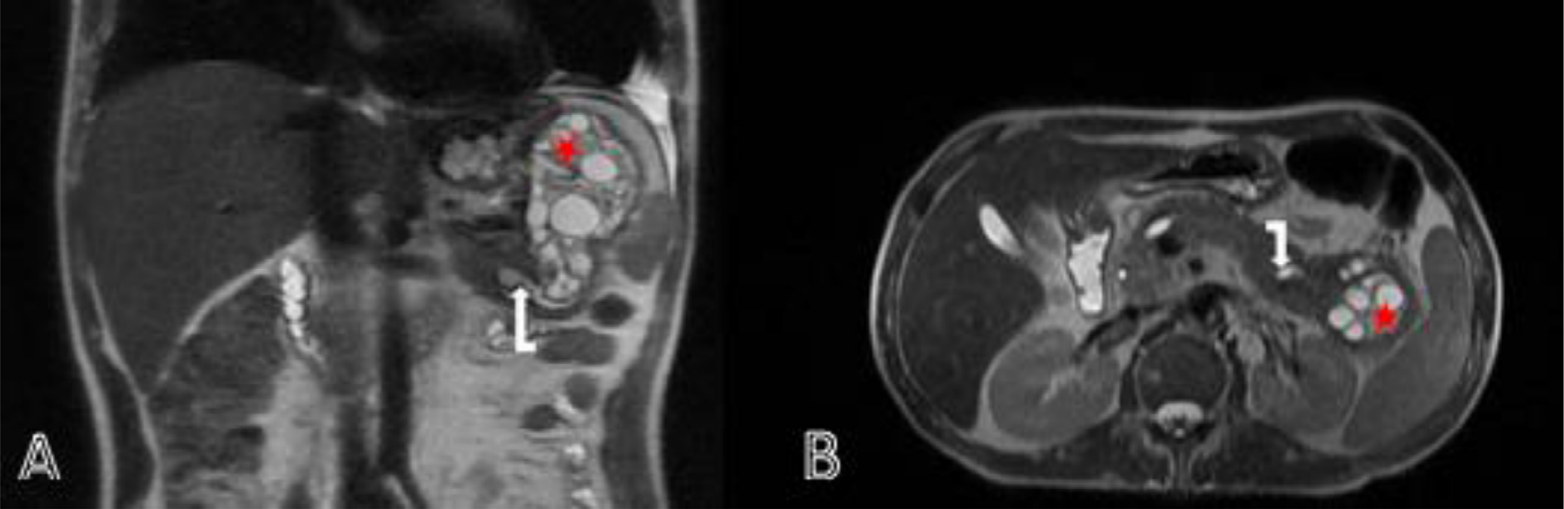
Coronal section (B) axial section of a Bili-MRI showing multiple daughter cysts inside the pancreatic cystic mass (red star), consistent with a type III hydatid cyst of the GHARBI classification. This cystic mass is fistulised in the Wirsung pancreatic duct (white arrow) causing a stage E pancreatitis.
